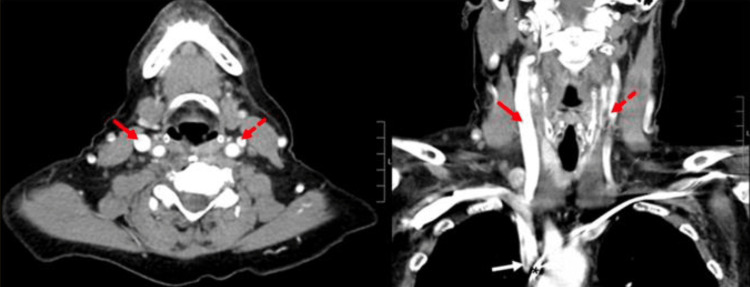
Axial (A) and coronal (B) CT neck with IV contrast, showcasing unilateral engorgement of the right internal jugular vein (solid red arrows) in comparison with the left internal jugular vein (dashed red arrows) secondary to stricture formation (solid white arrow) seen in the mid superior vena cava and right brachiocephalic vein adjacent to pacemaker leads (black asterisk). CT: computed tomography.
